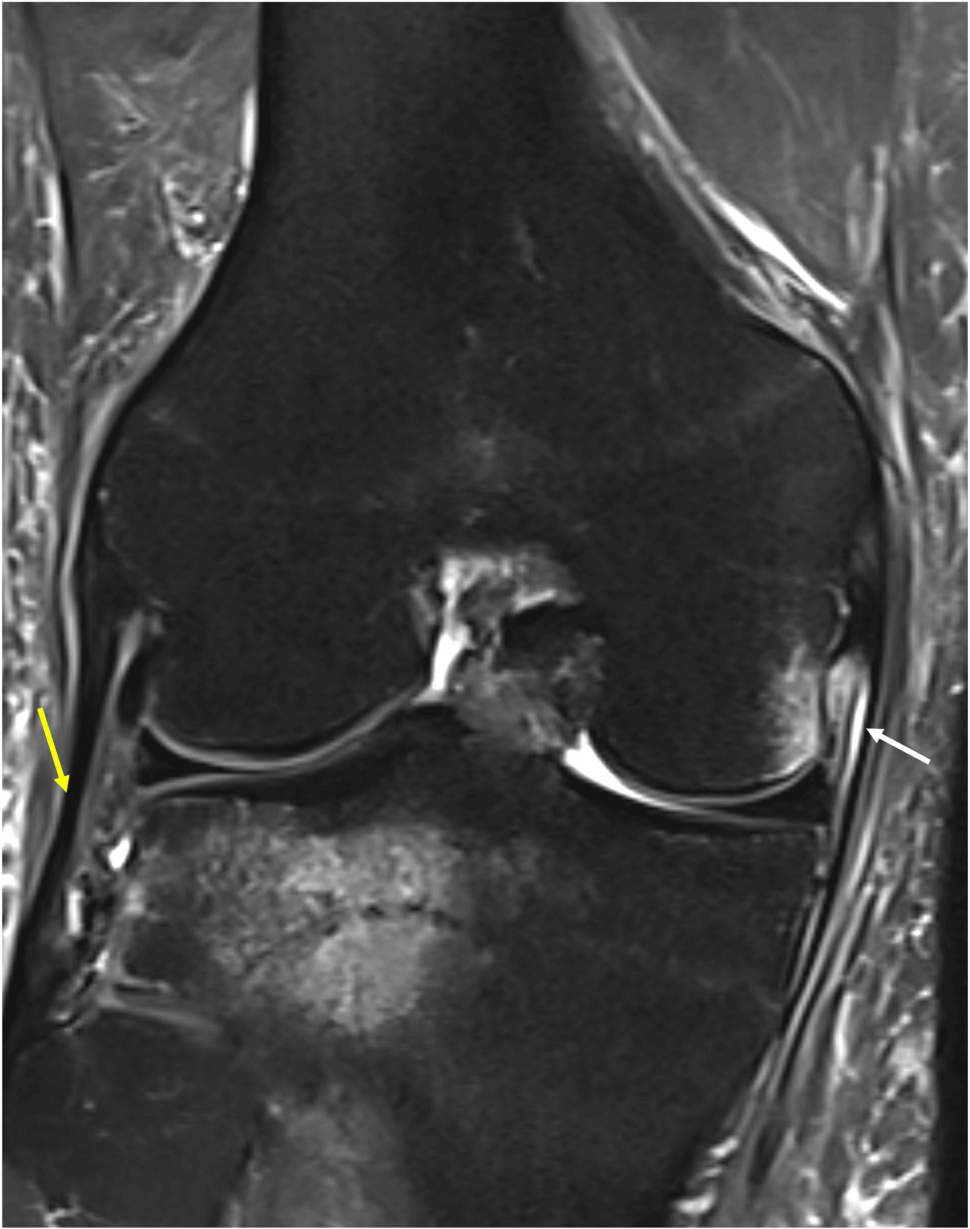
Coronal PDFS MRI showing grade 1 and 2 sprain in fibular (yellow arrow) and tibial (white arrow) collateral ligament respectively PDFS : proton density fat-saturated
